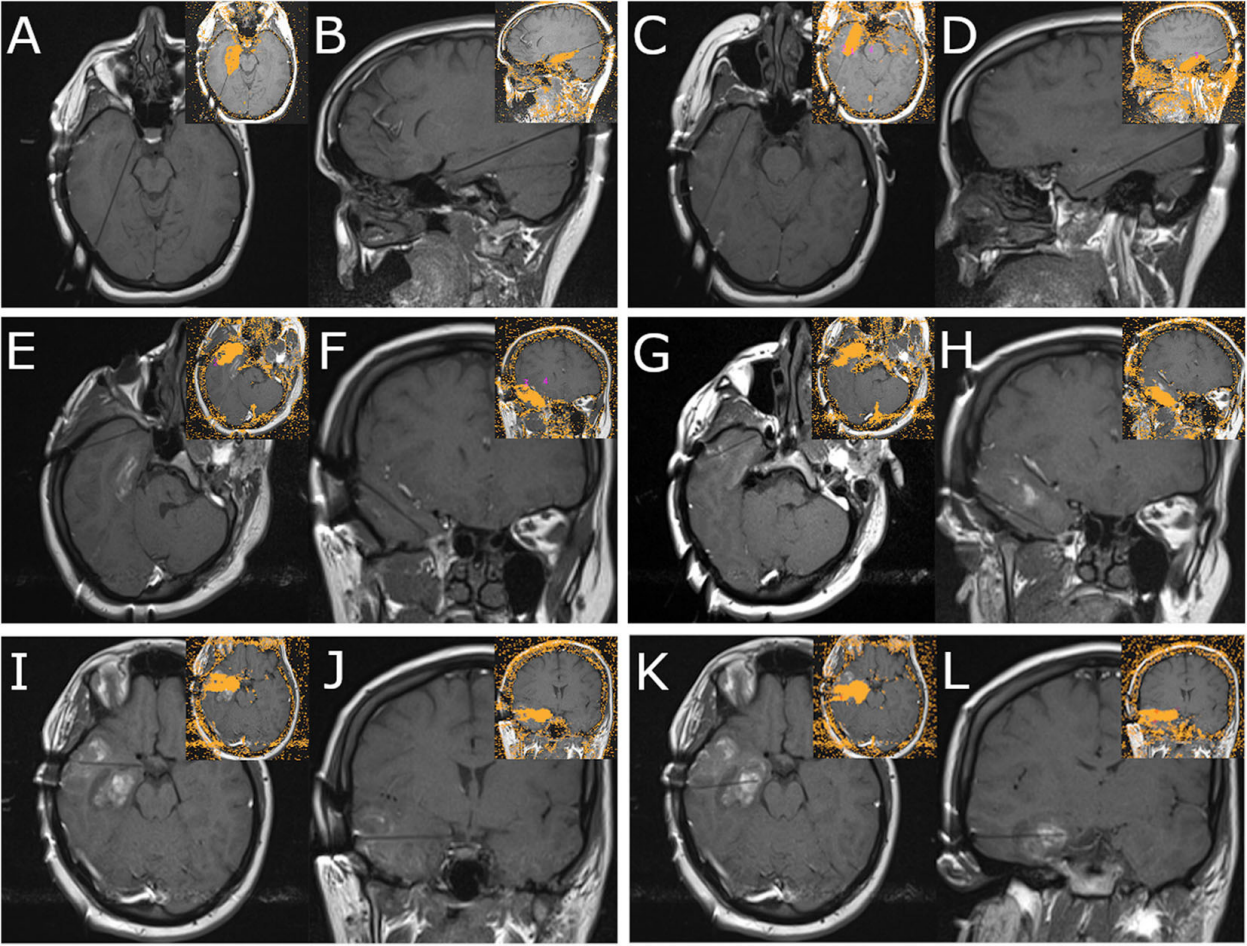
Panel demonstrates MRI images obtained during MRgLITT ablation of the right temporal lobe. Each panel demonstrates two orthogonal views along the laser fiber; inset images demonstrate the resultant ablation volume as determined by the Visualase system for each trajectory (Medtronic). (A,B) Panel demonstrates mesial hippocampal laser fiber trajectory. (C,D) Panel demonstrates medial temporal pole laser fiber trajectory. (E,F) Panel demonstrates superior lateral temporal pole laser fiber trajectory. (G,H) Panel demonstrates inferior lateral temporal pole laser fiber trajectory. (I,J) Panel demonstrates lateral approach to uncus laser fiber trajectory. (K,L) Panel demonstrates lateral approach to hippocampal body laser fiber trajectory.
